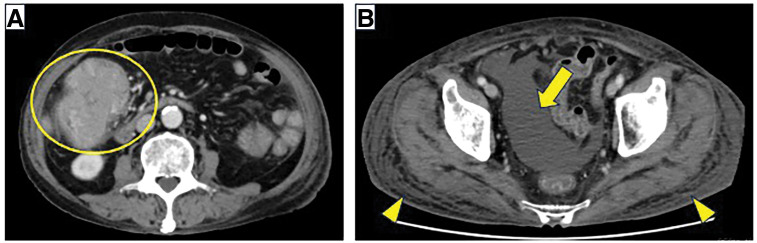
Contrast-enhanced abdominal CT. (A) A 58 × 78-mm enhancing mass lesion is observed in the ascending colon (yellow circle). (B) Ascites (arrow) and subcutaneous edema (arrowhead) are also noted.
